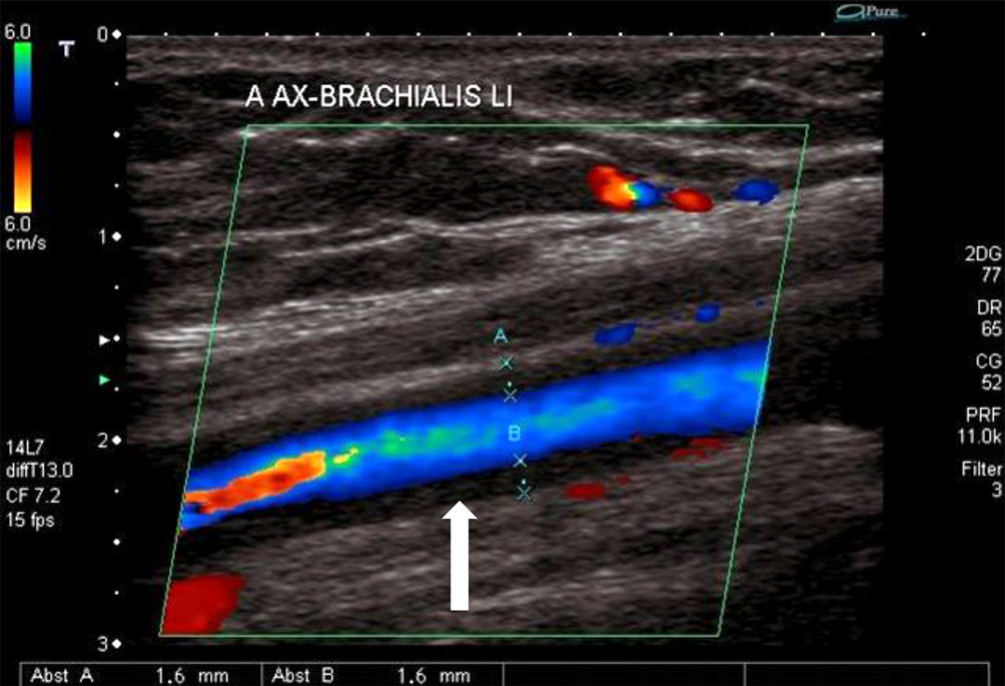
Takayasu arteritis with brachial artery involvement and typical halo (*arrow*) on color duplex sonography (courtesy of Dr. G. Herzog)
